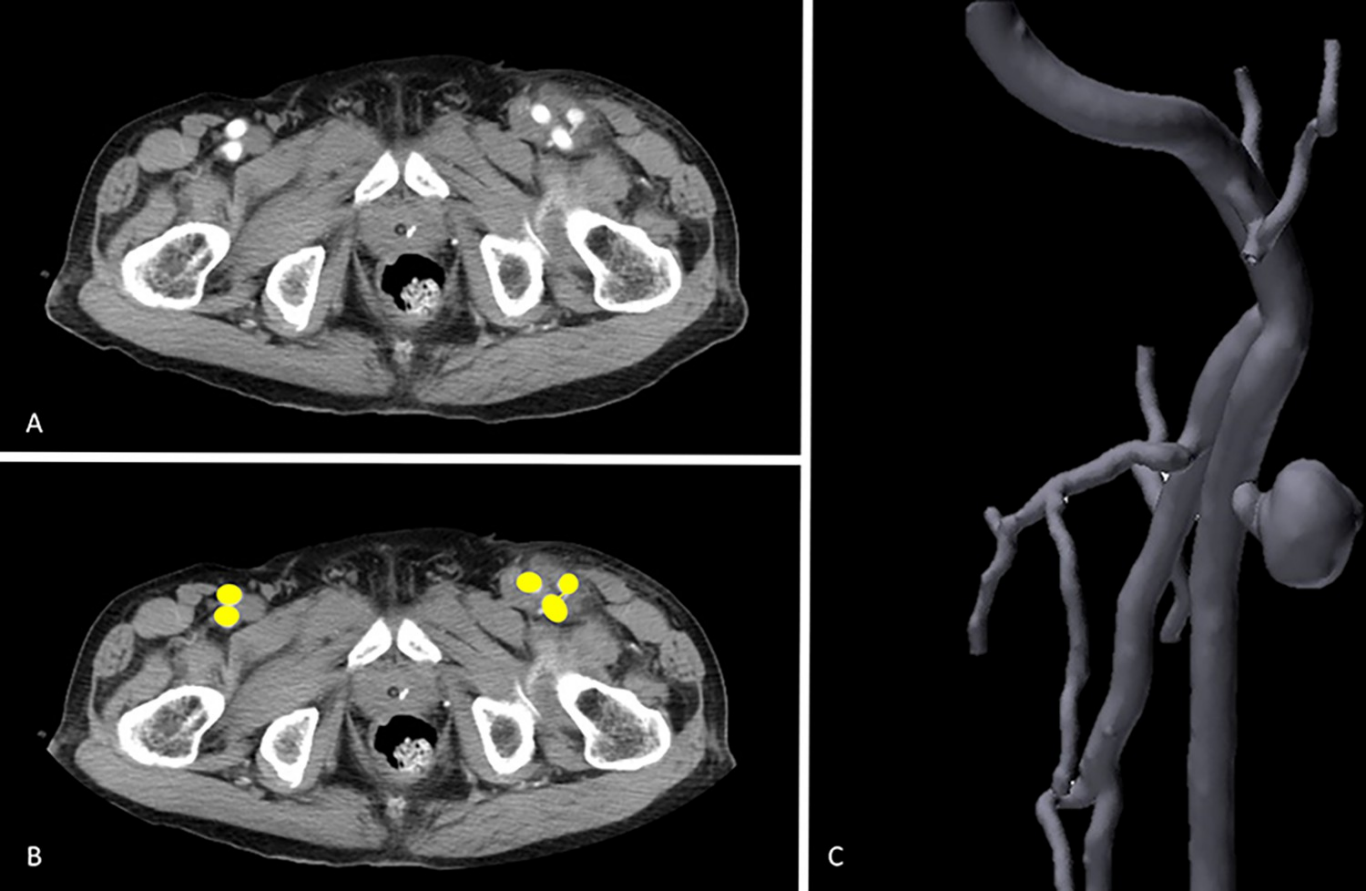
Pelvic Computed Tomography images(CT). The raw data of the pelvic CT with contrast (A) stored in the DICOM format was acquired to create the 3D model. (B) Then, the DICOM files are imported into Materialise Mimics 22.0 software where relevant arterial anatomy is segmented. (yellow) (C)Next the segmented model is exported to Materialise 3-Matic to create mesh representations of the individual parts or volumes to be printed.
